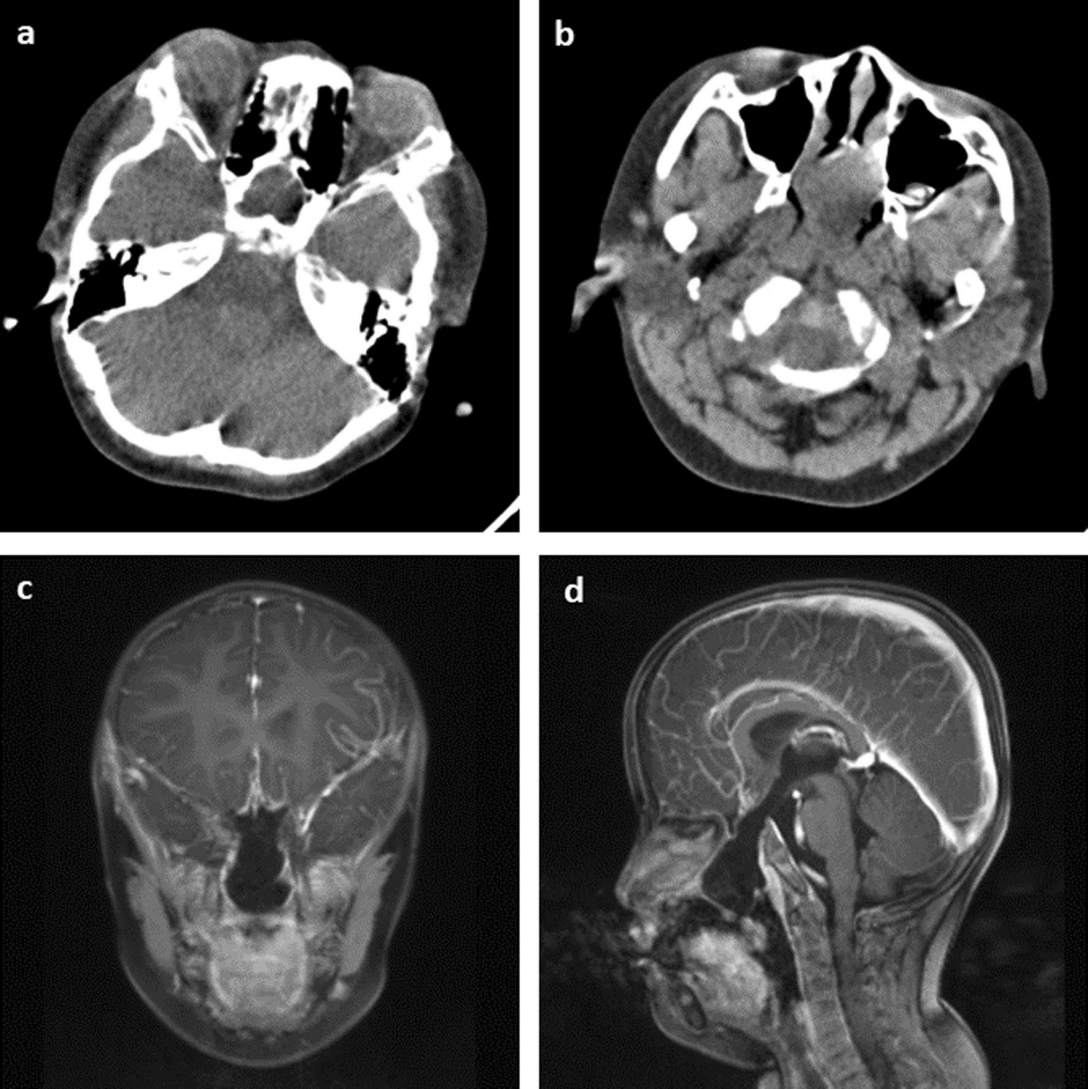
Demonstration of MRI and CT scan in trans-sellar encephalocele diagnosis. a, b CT scan showing the bony defect at the sellar region with a prominent encephalocele protruding into the nasopharynx. c, d Coronal and sagittal MRI view of the encephalocele showing that it merges with the nasal septum and obstructs the choana
